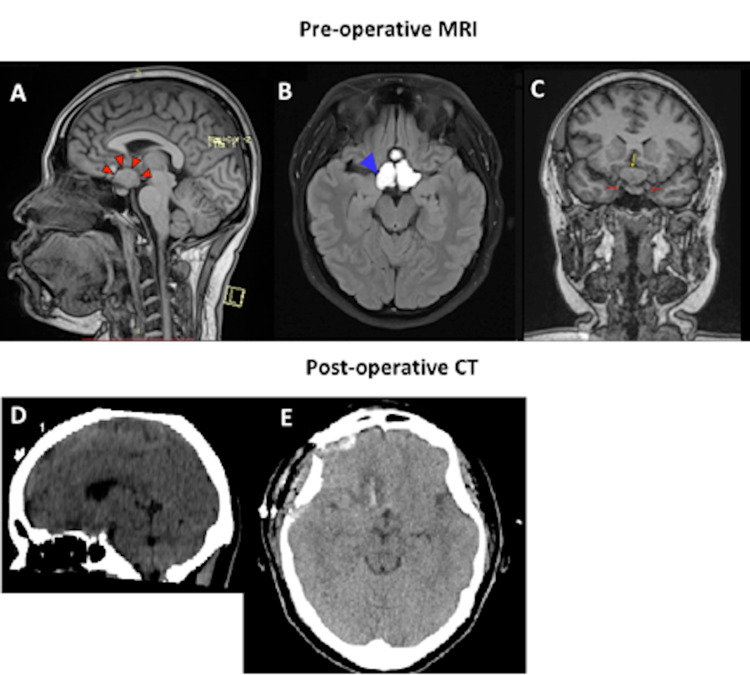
Pre-operative MRI brain showing: (A) Sagittal T1 showing suprasellar lesion (red arrowhead) compressing optic chiasma. The normal pituitary gland is seen, with infundibulum stretched posteriorly by the tumour; (B) Axial FLAIR image showing hyperintense suprasellar mass (blue arrowhead); (C) Coronal T1W showing suprasellar mass (yellow arrow) indenting both ICA (red arrow). Post-operative CT brain showing: (D and E) Plain CT axial and sagittal showing evidence of craniotomy with post-operative changes. Suprasellar mass not visualized. ICA: Internal carotid artery; FLAIR: Fluid-attenuated inversion recovery.
